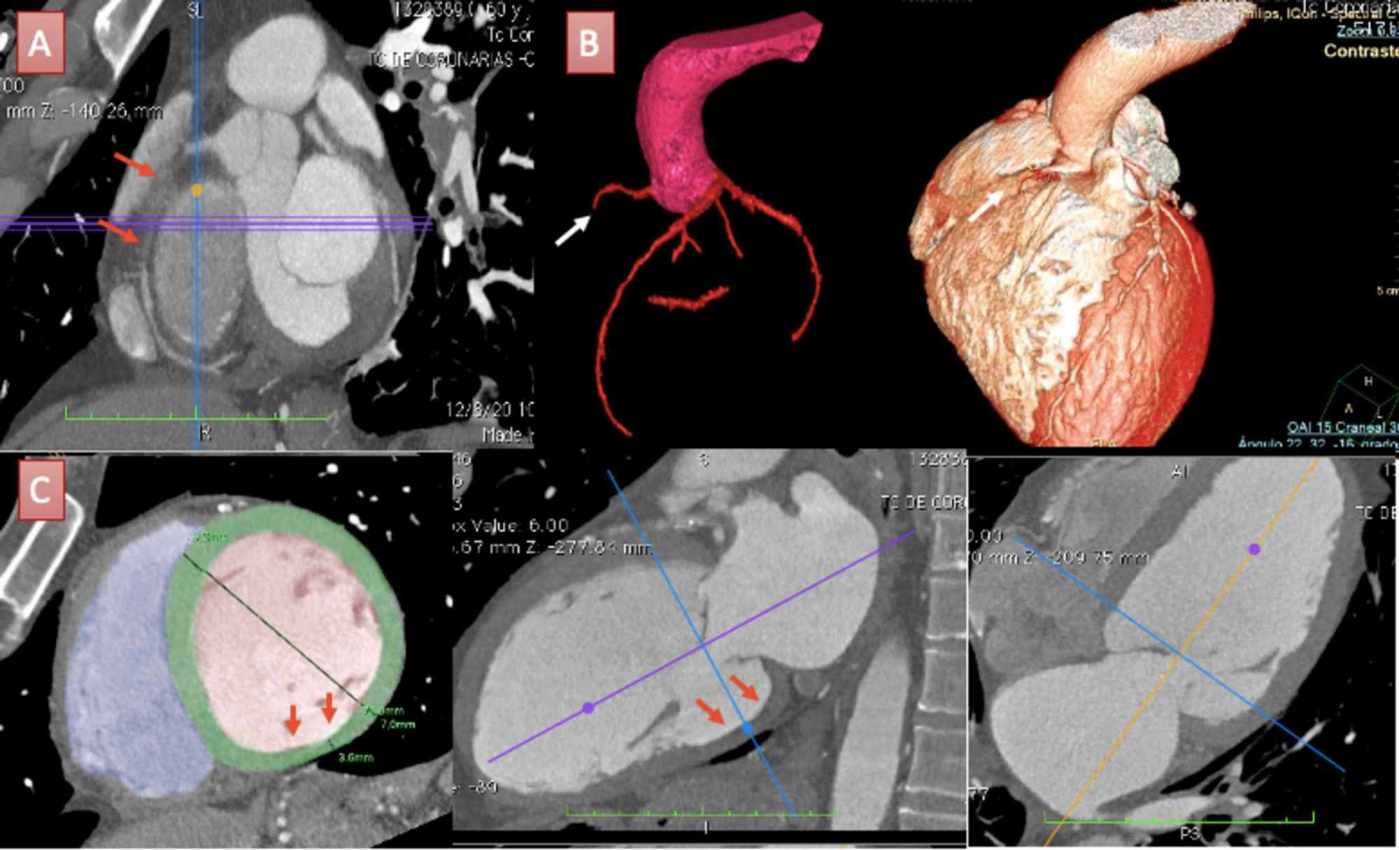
Coronary computed tomographic angiography findings of the patient. A: Multiplane reconstruction of the right coronary artery (RCA) showing total occlusion at the proximal segment (red arrows). B: Tri-dimensional (3D) volume rendering of coronary arteries and cardiac volume showing total occlusion of the RCA (white arrows) and distribution of coronary arteries from an anteroposterior view of the heart. C: Multiple planes reconstruction of the left ventricle (LV). LV short-axis view (bottom left) estimated an end-diastolic LV diameter of 70 mm consistent with a severe dilatation and denoted an inferobasal wall thickness of 3.6 mm (red arrows). LV long-axis two and four-chamber views (bottom middle and right) consistent with inferior basal LV thinned wall (red arrows).
